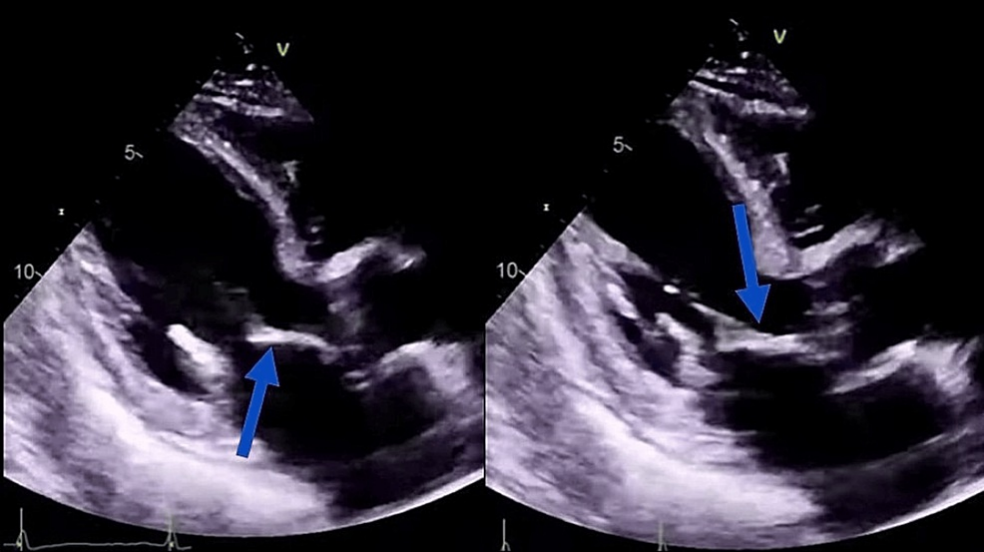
Echocardiogram showing vegetation at tricuspid valve (blue arrow)
